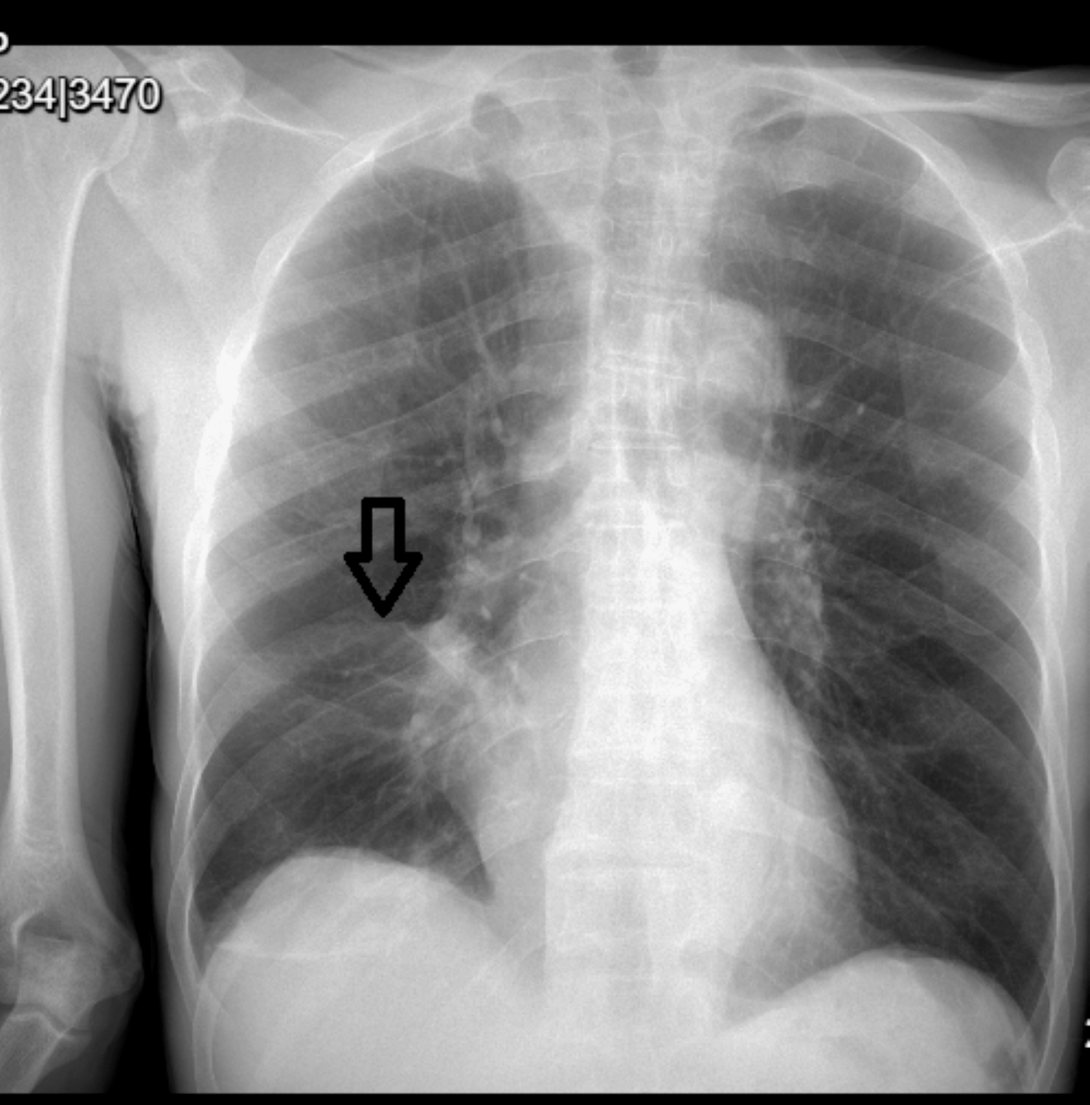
Chest X-ray showing partial atelectasis of the right middle lobe
